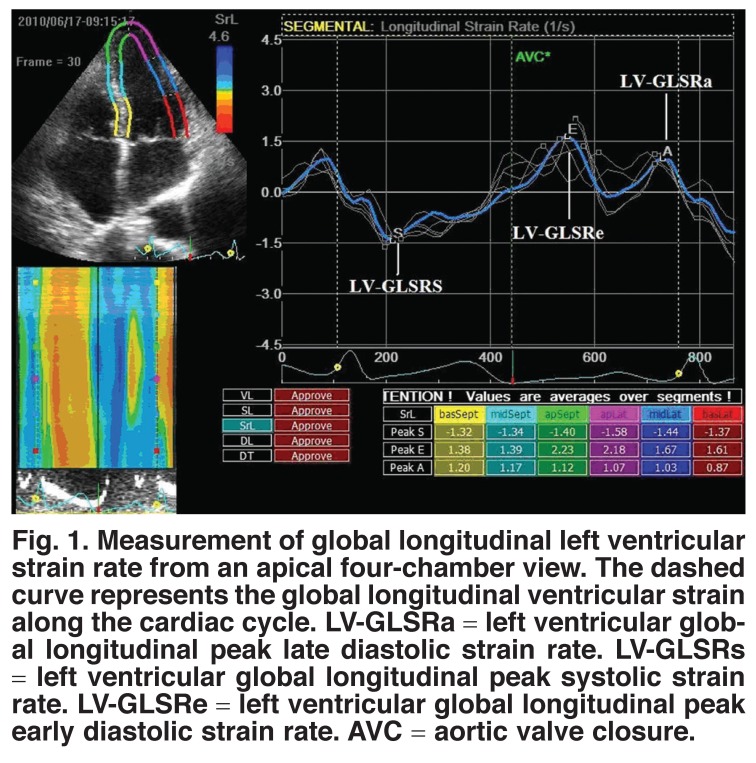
Measurement of global longitudinal left ventricular strain rate from an apical four-chamber view. The dashed curve represents the global longitudinal ventricular strain along the cardiac cycle. LV-GLSRa = left ventricular global longitudinal peak late diastolic strain rate. LV-GLSRs = left ventricular global longitudinal peak systolic strain rate. LV-GLSRe = left ventricular global longitudinal peak early diastolic strain rate. AVC = aortic valve closure.
